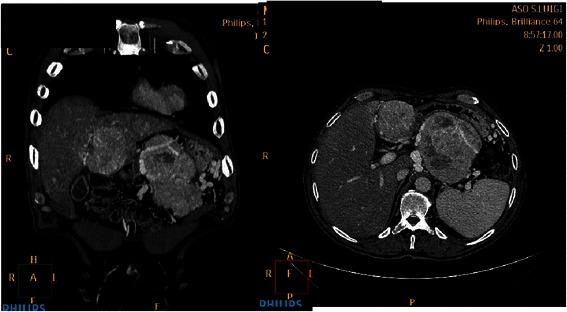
Contrast computed tomography (CT) images of a patient with paraneoplastic cerebellar degeneration due to metastatic pancreatic neuroendocrine tumour
